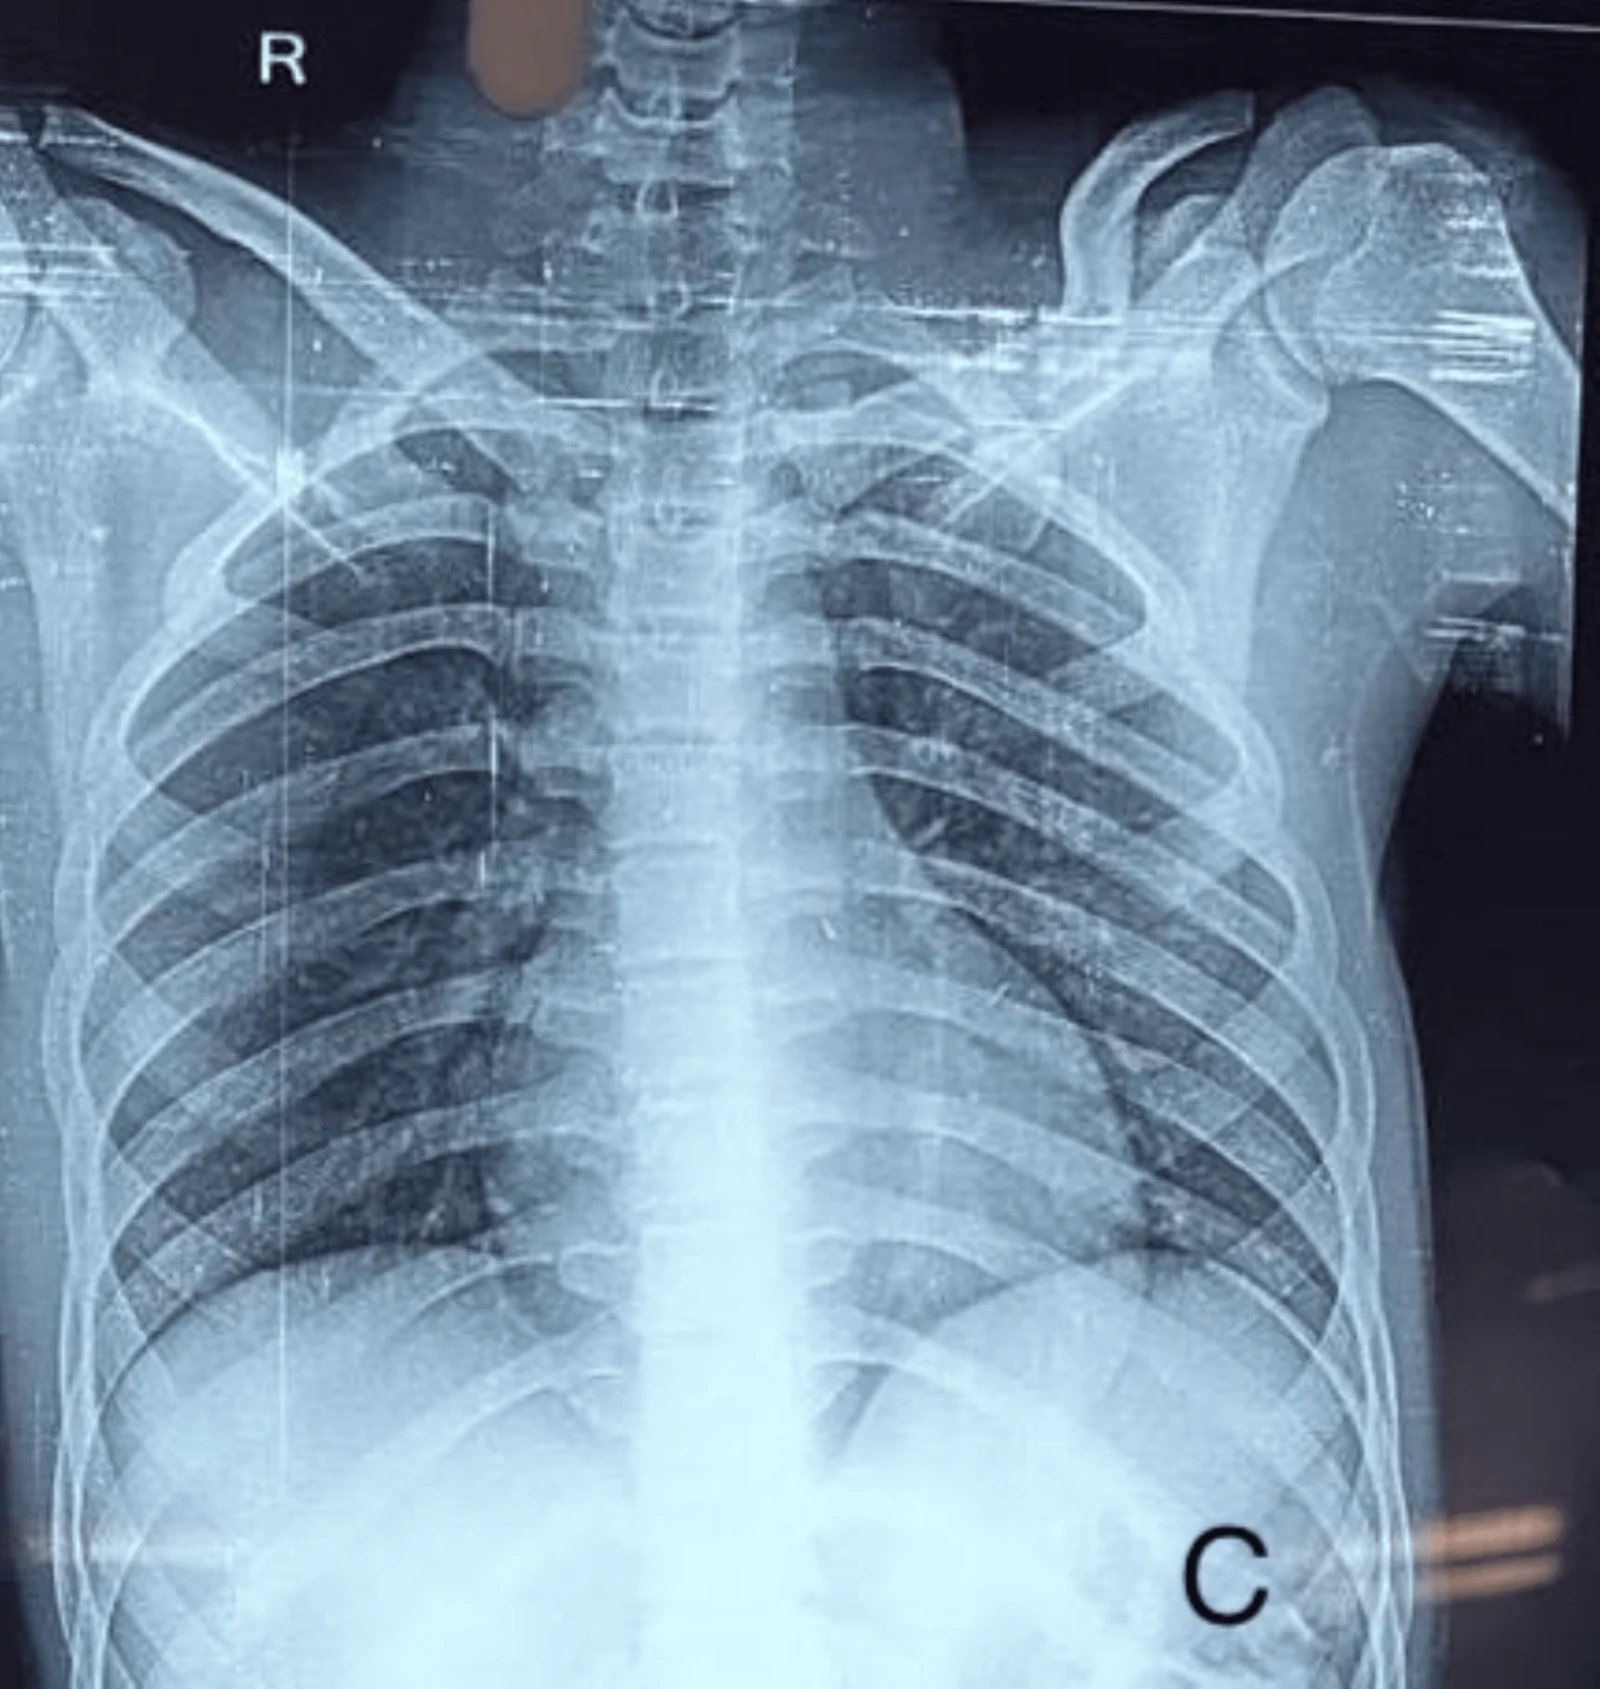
AP chest radiograph of a 19-year-old man showing a displaced fracture of the mid-shaft of the left clavicle. AP: anteroposterior
